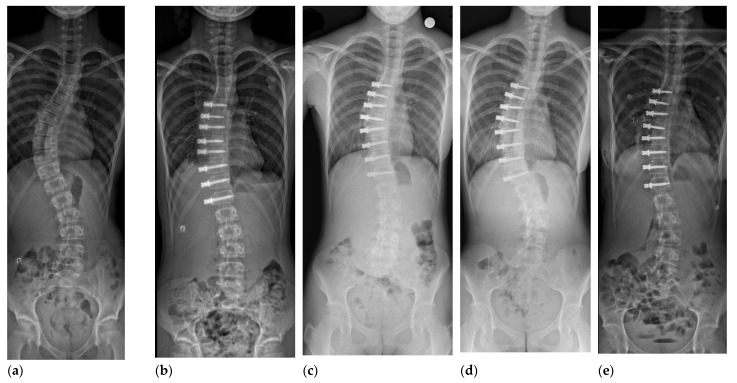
12 year-old girl, Sanders 2, Lenke 1 AN curve of 50 degrees (a). First erect postoperative radiograph (b), 1 year follow-up (c), 30 month follow-up demonstrating splaying of the screw heads at multiple levels and progression of the deformity (d), and after revision surgery (Sanders 6) for replacement of broken tether (e).
